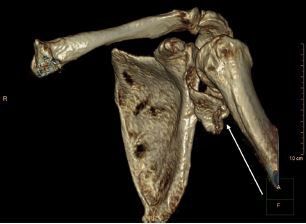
Computed tomography three-dimensional reconstruction of the shoulder showing proper articulation of humeral head within the glenoid fossa at the correct level. Fracture fragment noted by the arrow.
